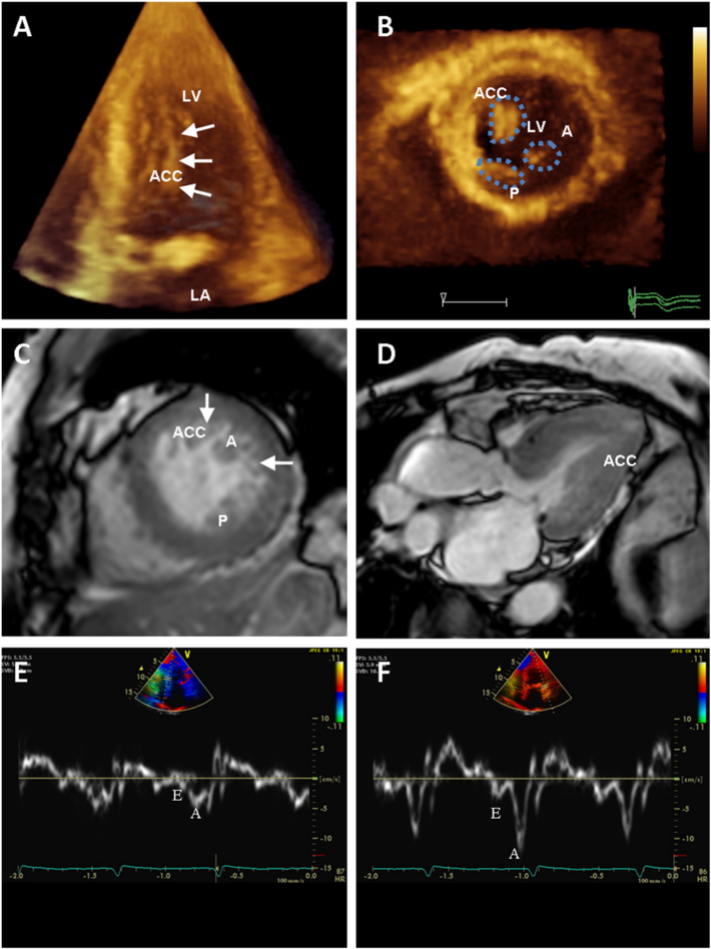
Real 3D echocardiography, MRI, and tissue doppler imaging. (A) 2D echocardiogram in the short-axis section of the left ventricle demonstrated uncommonly enlarged papillary muscles (A: anterolateral papillary muscle, P: posteromedial papillary muscle, ACC: accessory papillary muscle). (B) Real time 3D Echocardiography demonstrated that LV mid-cavity was almost obliterated during end-systolic phase from five chamber view (Additional file 1, movie 1). A: anterolateral papillary muscle, LA: left atrium, LV: left ventricle. (C) Contrast-enhanced MRI from LV short-axis showed two large papillary muscles as well as adjacent abundant muscular trabeculae producing mid-cavity obstruction during systolic phase. (D) MRI from long-views showed anterolateral papillary muscle squeezing left ventricular outflow tract against LV septum during systolic phase. High temporal resolution Tissue Doppler Imaging showed markedly diminished diastolic mitral annulus relaxation velocities, 1.8 cm/sec for basoseptal (E) and 4.3 cm/sec for basolateral segments (F).
